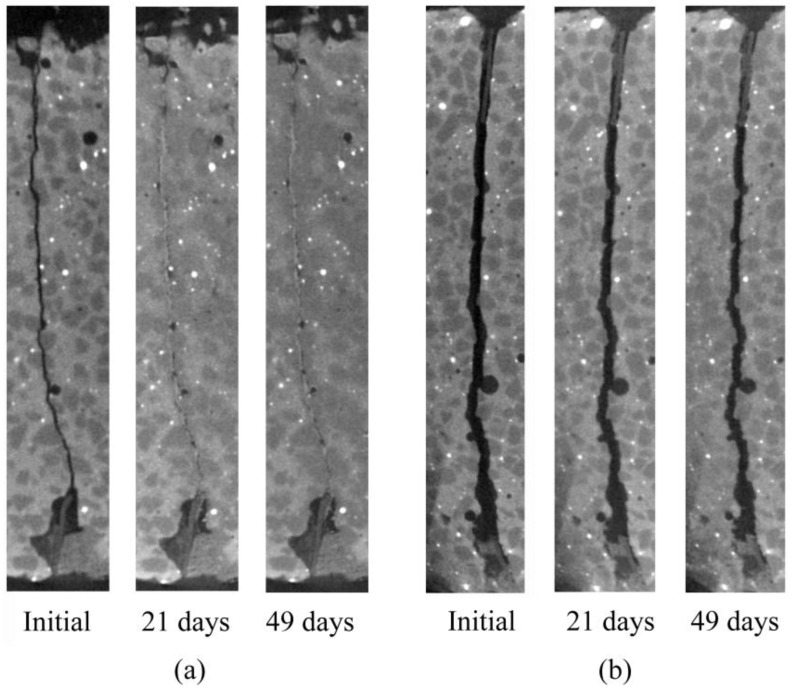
Comparison of CT images for a particular section near (a) End-I and (b) End-II. Height and width of each image are 14 and 2 mm, respectively.
